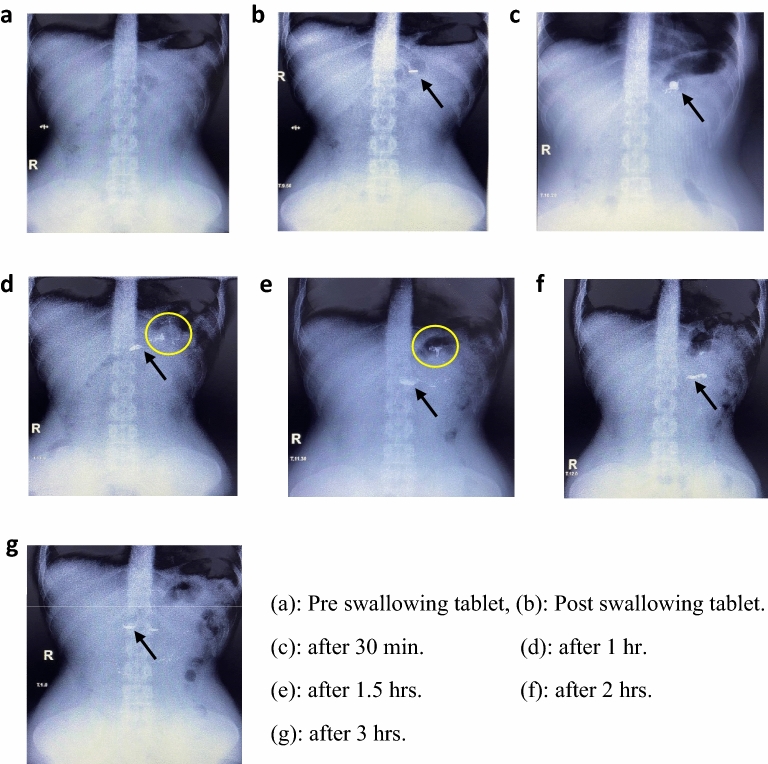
Radiographic images of F7 at different times after oral administration.
